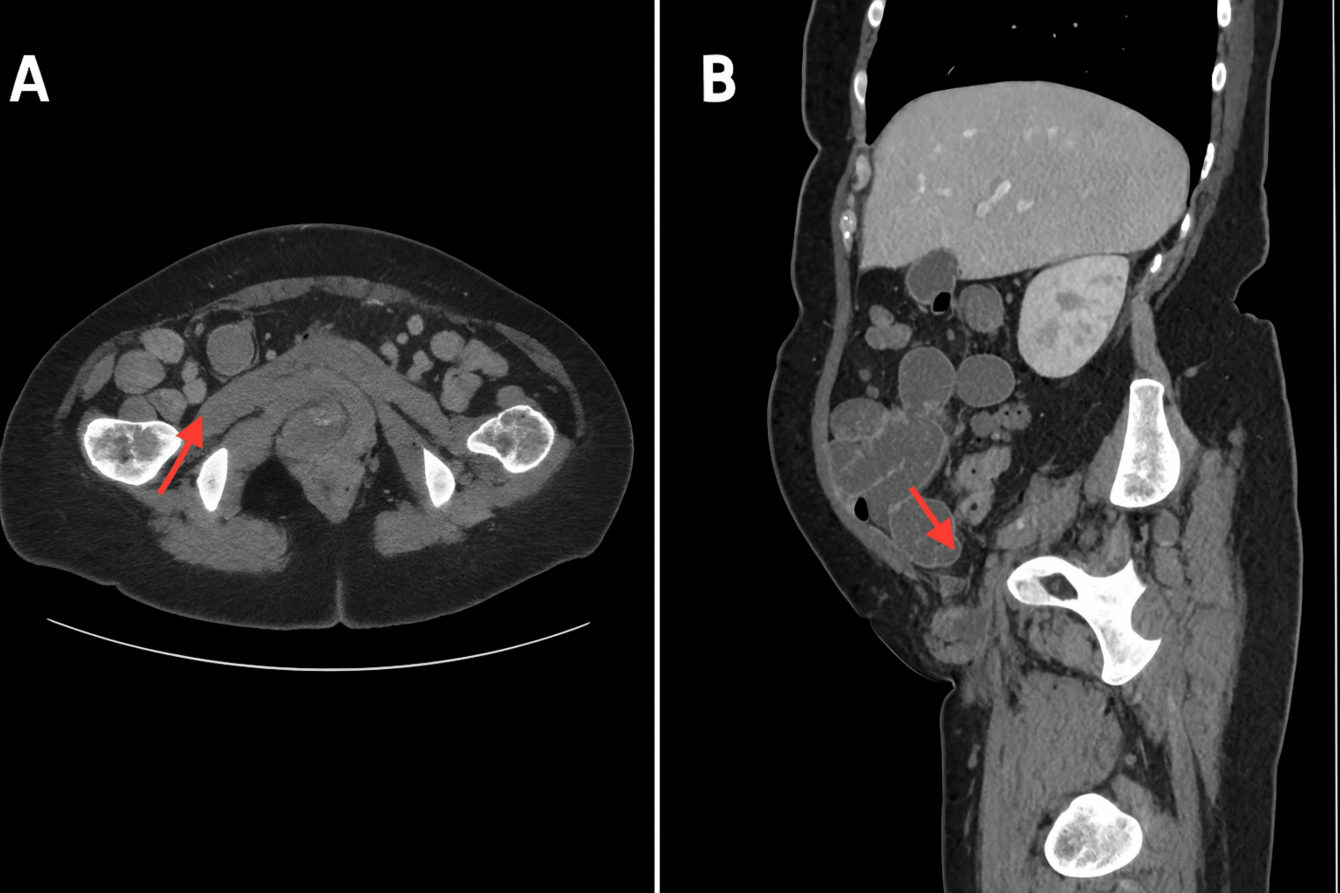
Results of abdominal CT investigation showing evidence of obstruction at the right femoral ring on the coronal (A) and sagittal (B) views Abdominal CT at presentation (coronal view A and sagittal view B) showing evidence of small bowel obstruction at the right femoral canal (arrow), consistent with a strangulated femoral hernia
